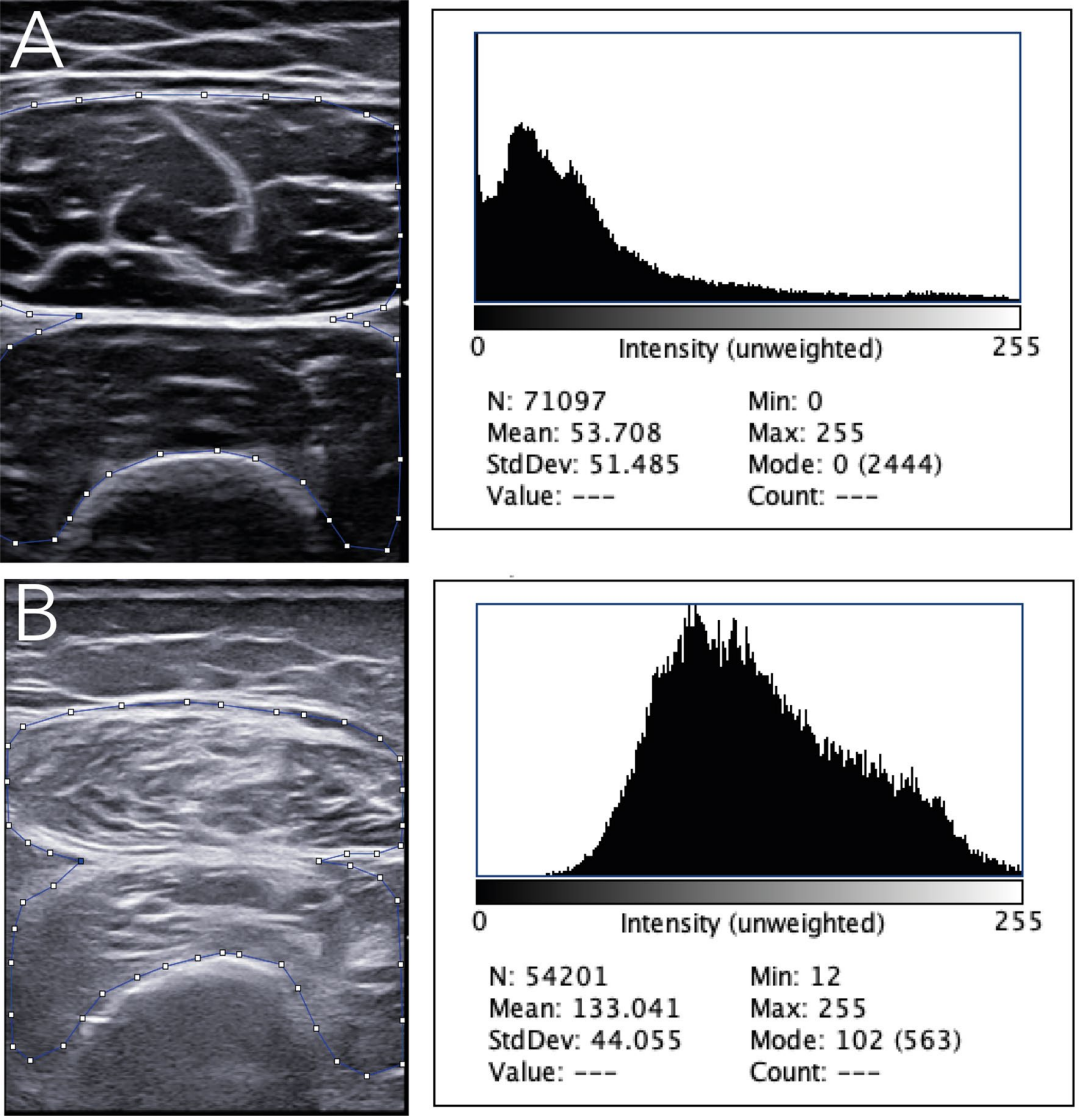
Muscle echogenicity in grayscale and histographic analysis in a healthy subject (a) compared to a patient with systemic sclerosis (b). An ultrasound transverse scan image of the rectus femoris (rf) and vastus intermedius (vi) showing increased muscle echogenicity in the patient with systemic sclerosis in comparison with the healthy subject (i.e., grade III of the Heckmatt scale, marked increased muscle echo with reduced bone echo vs grade I of the Heckmatt scale, normal hypoechoic muscle) [76]. The small squares and lines indicate the region of interest for grayscale image analysis with histograms
